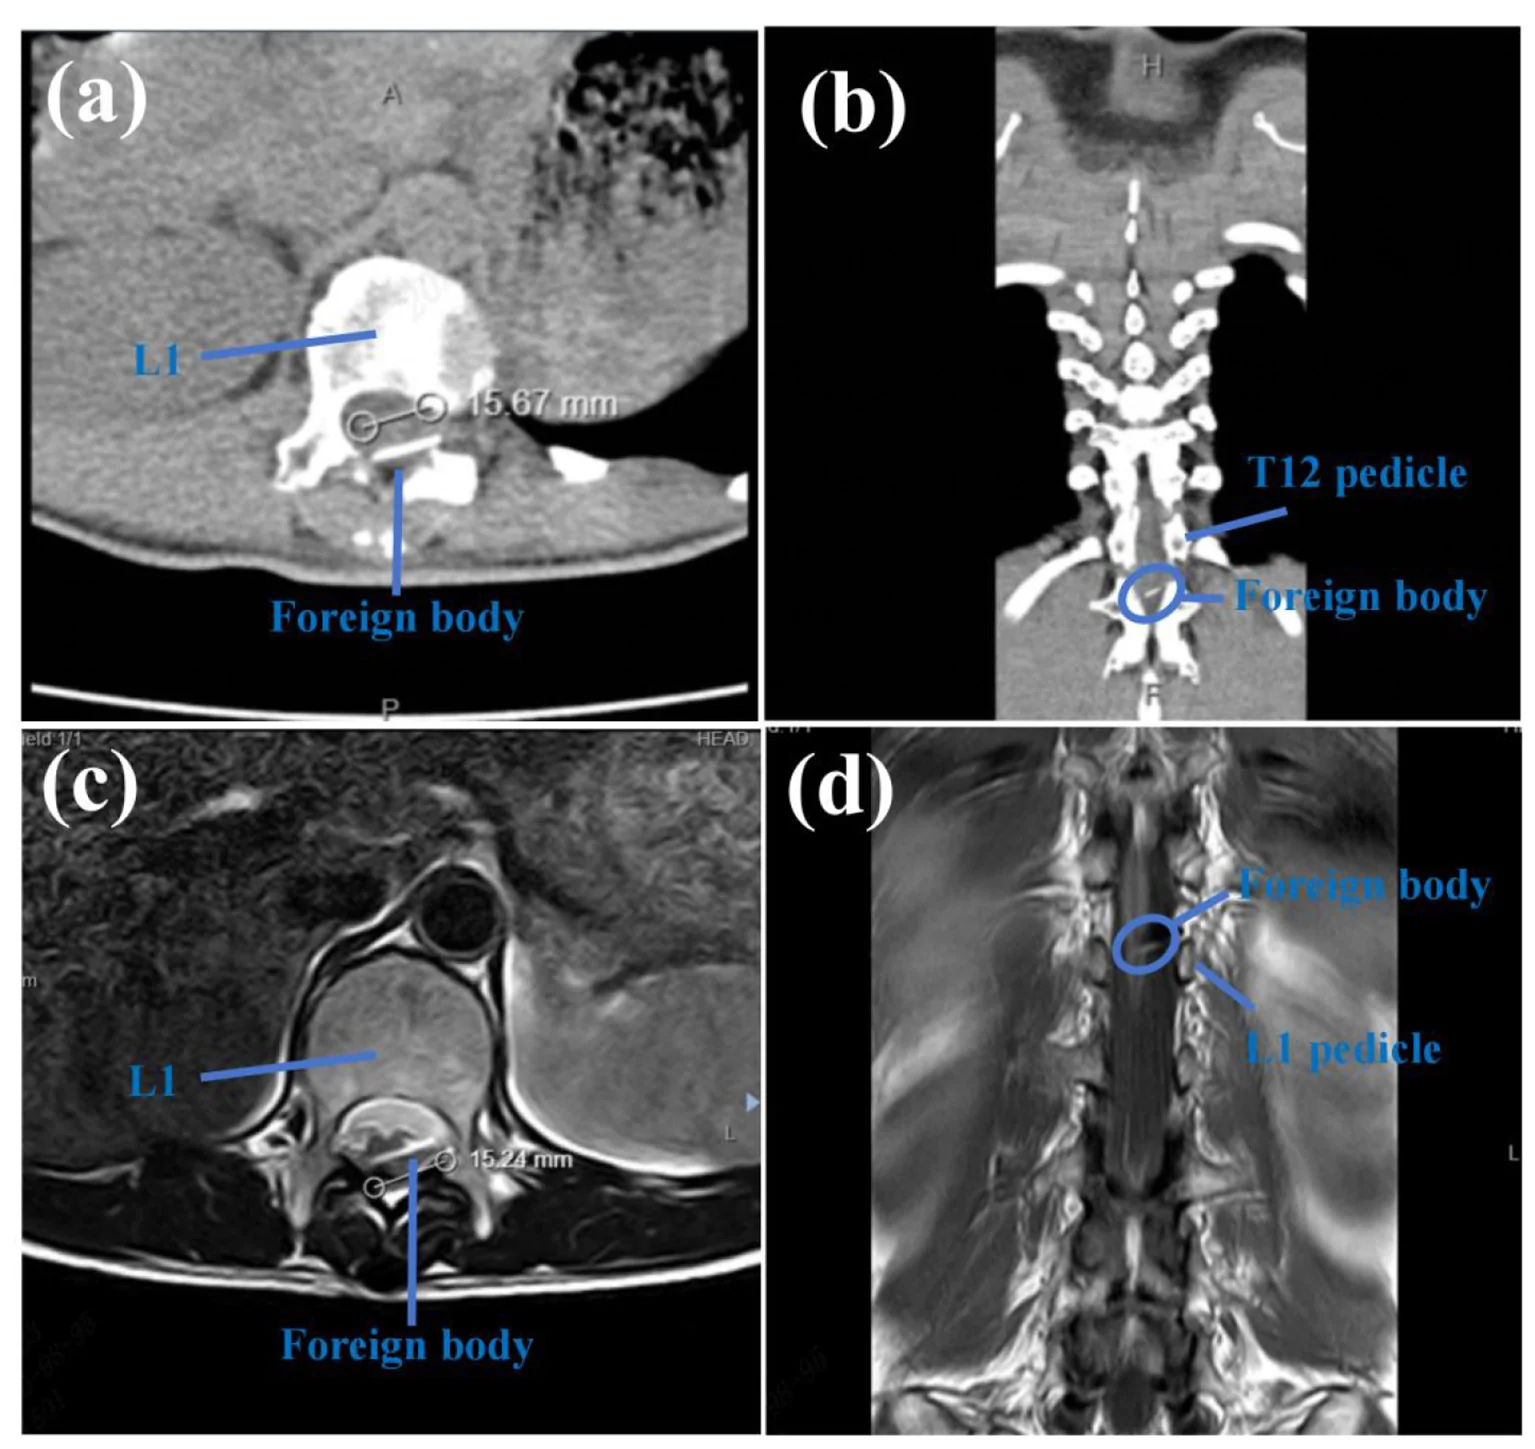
Pre-operative spinal imaging demonstrating the retained metallic foreign body. (a) Axial and (b) coronal CT images showing a high-density linear metallic foreign body at the T12-L1 level, measuring 15.67 mm in length. (c) Axial and (d) coronal T2-weighted MRI confirming the precise intradural location of the lesion, measuring 15.24 mm in length. Vertebral levels are explicitly labeled to facilitate anatomical localization.
